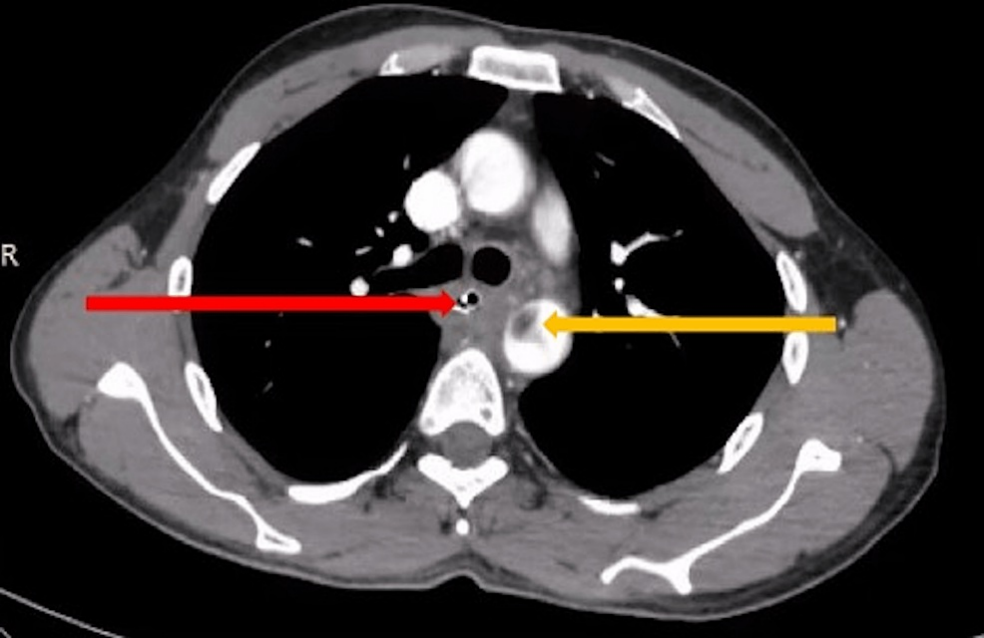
Contrast-enhanced CT thorax showing foreign body in the esophagus and thrombus in adjoining aorta The red arrow indicates a foreign body in esophagus. The yellow arrow indicates a thrombus in descending thoracic aorta.
